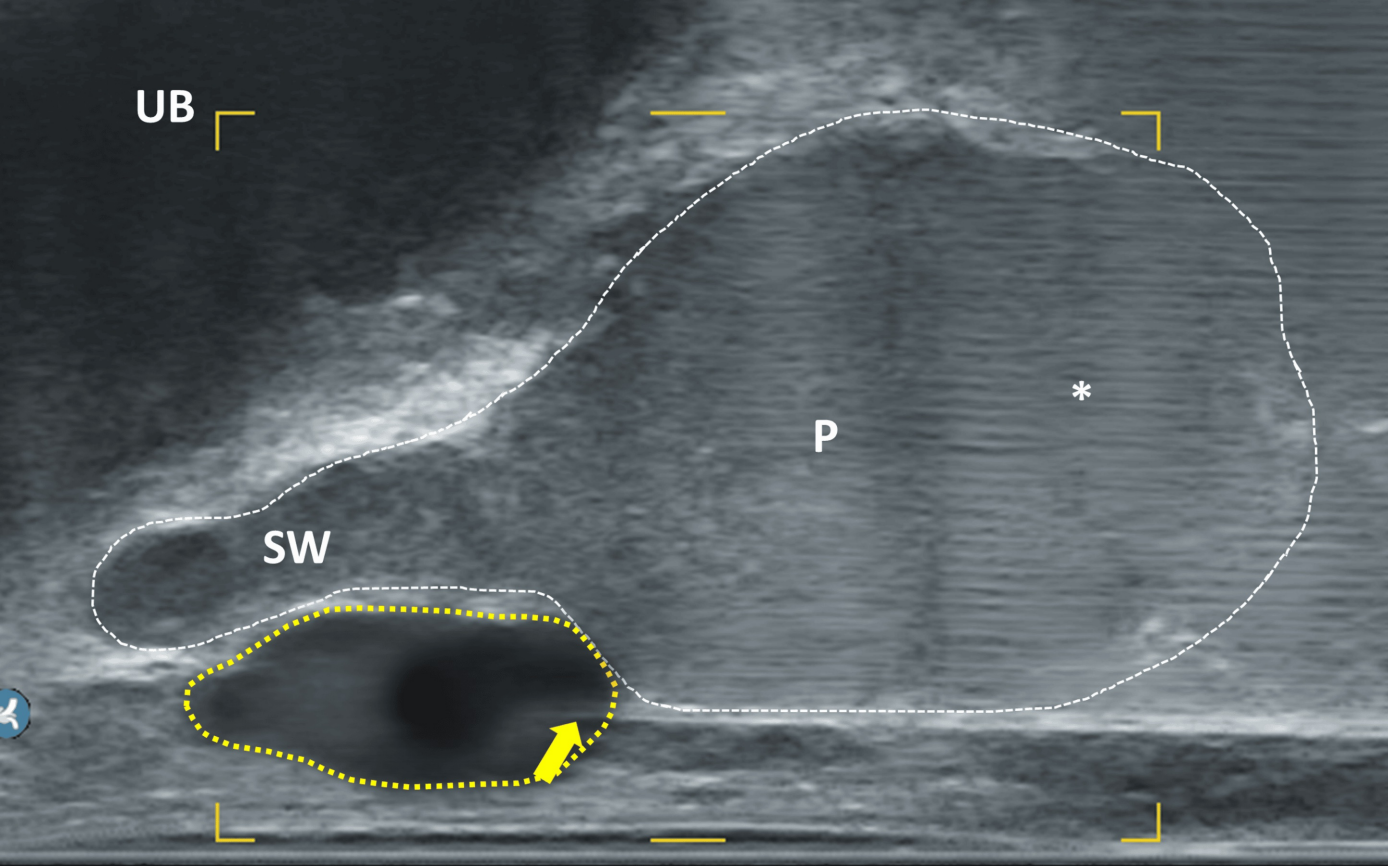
Sagittal section of the prostate UB: urinary bladder; P: prostate; SV: seminal vesicle; area enclosed by yellow dotted line: local anesthetic as echofree cushion below the seminal vesicle; yellow arrow: needle tip; *artifact due to the ultrasound extinction by the needle Image Credit: Toni Franz, corresponding author
